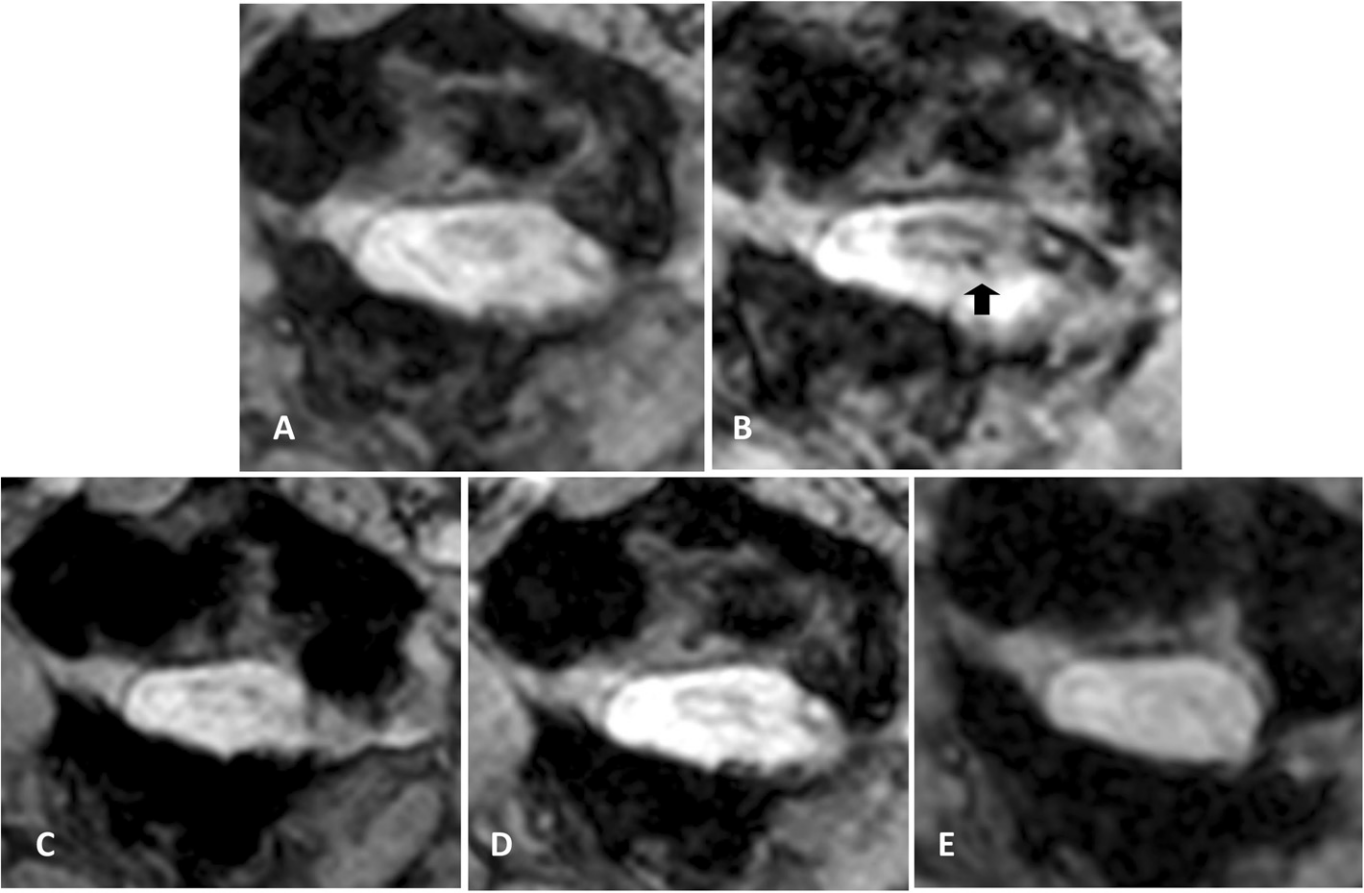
Timeline of axial T2-star echo5 magnetic resonance imaging scans of his cervical spine at the C1 level on pre-transplantation (A), two days (B), two weeks (C), one month (D) and seven months (E) after stem cell transplantation. The axial T2-star scan taken two days after stem cell transplantation (B) showed focal hyposignal intensity of tagged stem cells in the surface of the pathological upper cervical cord (arrow) at the axial T2-star scan taken two weeks after stem cell transplantation showed fading of the focal hyposignal intensity of tagged stem cells (C), which disappeared on the scans taken at one and seven months.
